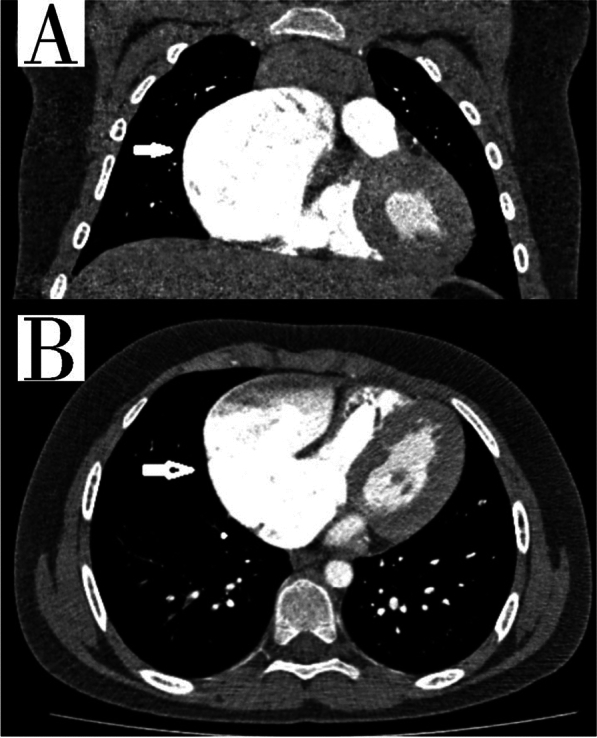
CT scan of the chest organs. A Frontal section, the arrow shows the aneurysmal expansion of the RA, B axial section, the arrow shows the aneurysmal expansion of the RA
